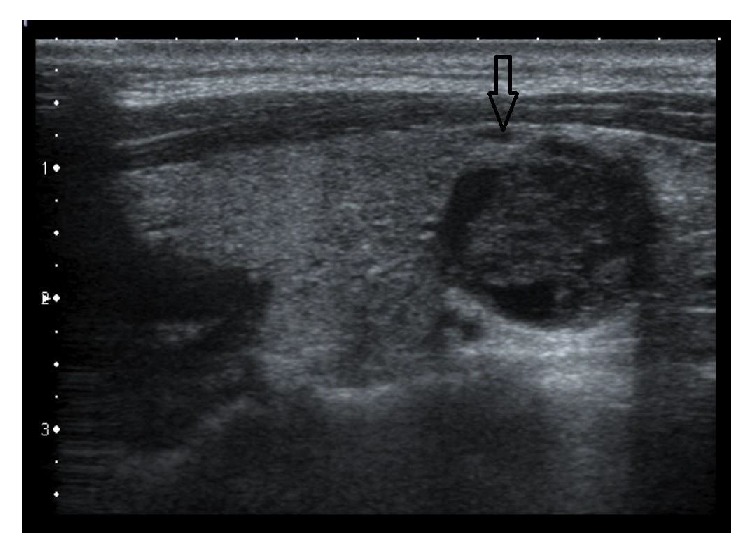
Ultrasound neck showing a well-defined hypoechoic parathyroid adenoma (arrow).
